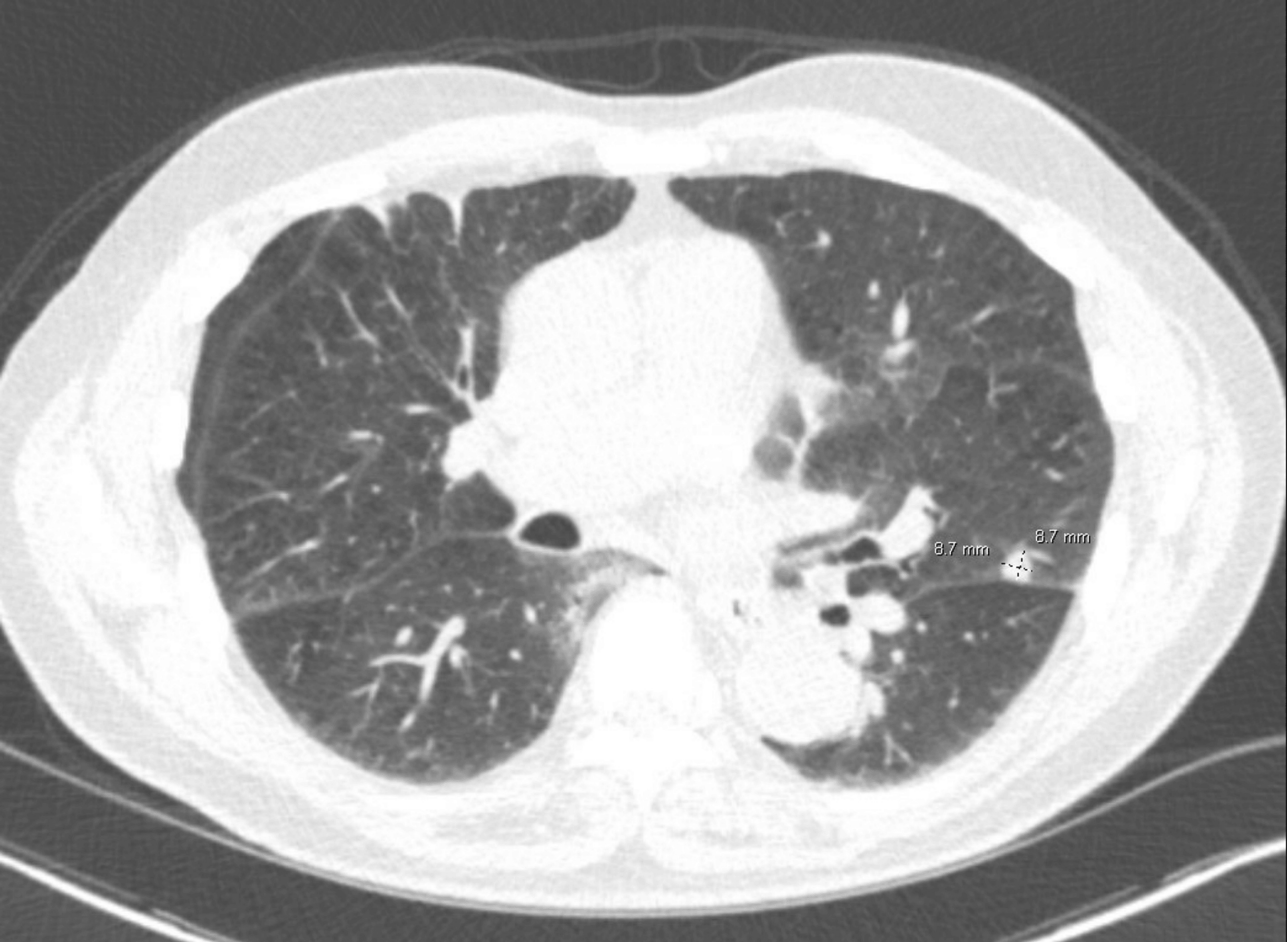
CT Chest with 9mm left upper lobe lung nodule
